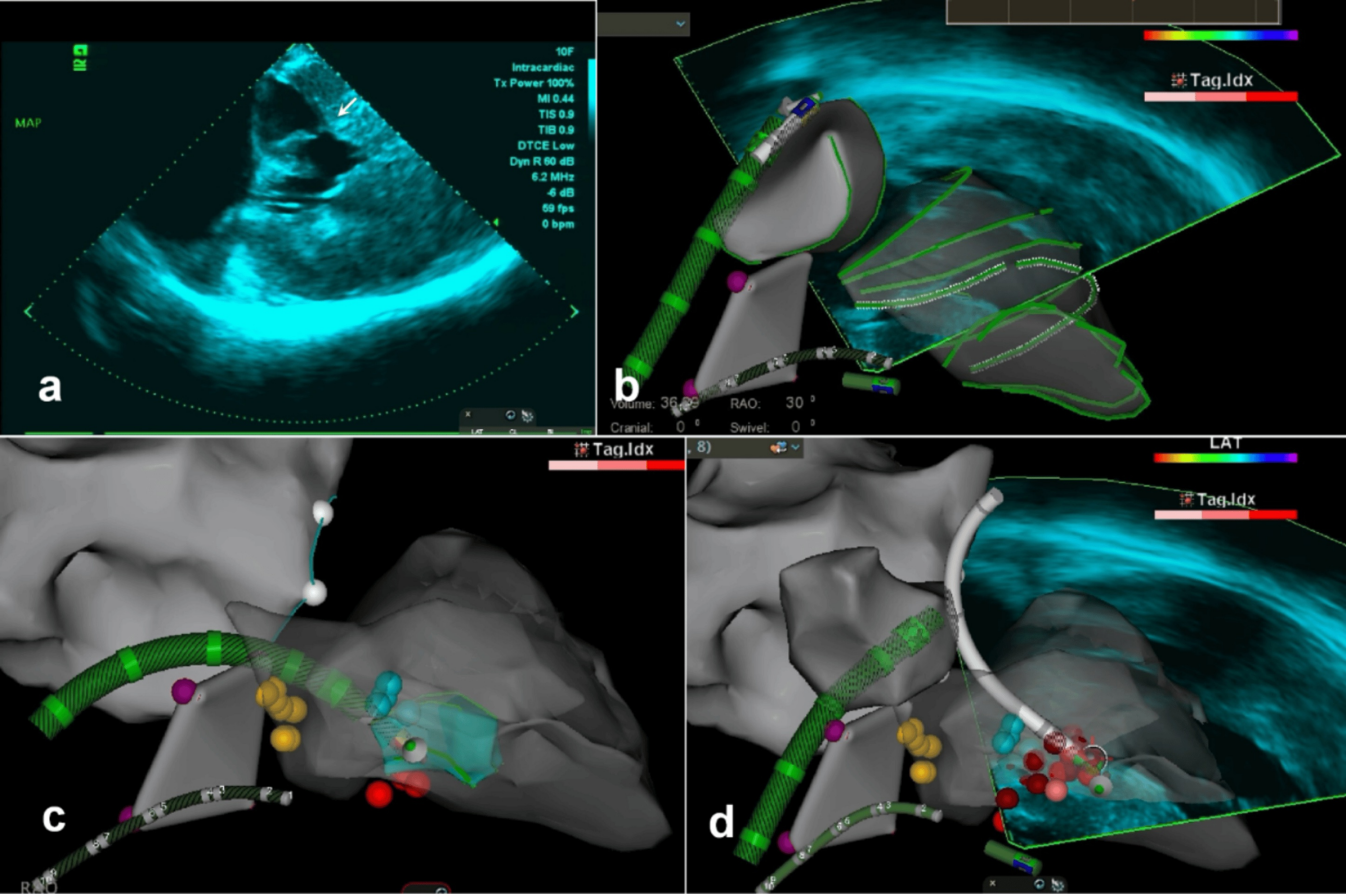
ICE-guided ERASH with the CARTO3 and CARTOSOUND system a) SAM (white arrow) on ICE; b) left ventricular mapping procedure; c-d) the ablation procedure was performed using both retrograde transaortic and transseptal approaches. The red dots show the ablation position; the yellow dots show the bundle of His; the purple dots show the tricuspid annulus; and the white dots show the mitral annulus. ICE, intracardiac echocardiography; ERASH, endocardial radiofrequency ablation for septal hypertrophy
